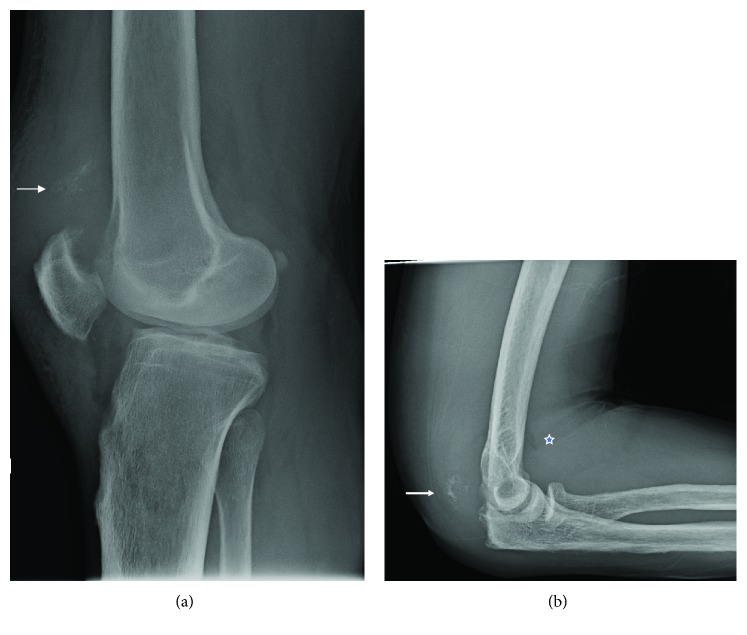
(a) Lateral X-rays of the right knee showing osseous flake (arrow) proximal to the low-riding patella, anterior tilt of proximal patella, and veiled aspect of the patellar tendon. (b) Lateral X-rays of the right elbow showing osseous flake (arrow) of the proximal olecranon and intra-articular effusion with typical sail sign (star).
